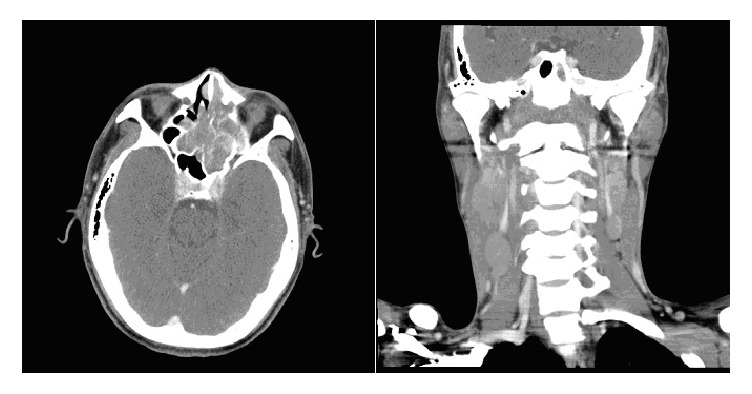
Contrast-enhanced CT of the head and neck reveals iso-enhancing masses in the left nasal cavity, nasopharynx, and paranasal sinuses, accompanied by bilateral neck lymphadenopathy.
